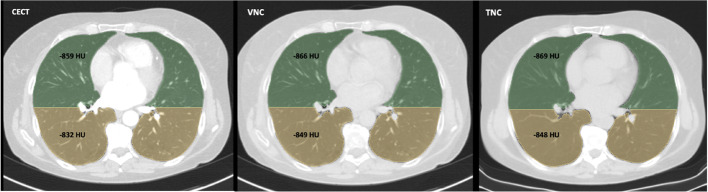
Axial CT scans of an exemplary patient: contrast-enhanced (left), virtual non-contrast (VNC) (middle), true non-contrast (TNC) (right); colored visualization of ventral and dorsal isovolumetric segmentation of the lung with annotations of the mean lung density (MLD) in HU. The example shows a greater increase in density at higher baseline HU and only minimal differences between VNC and TNC images
